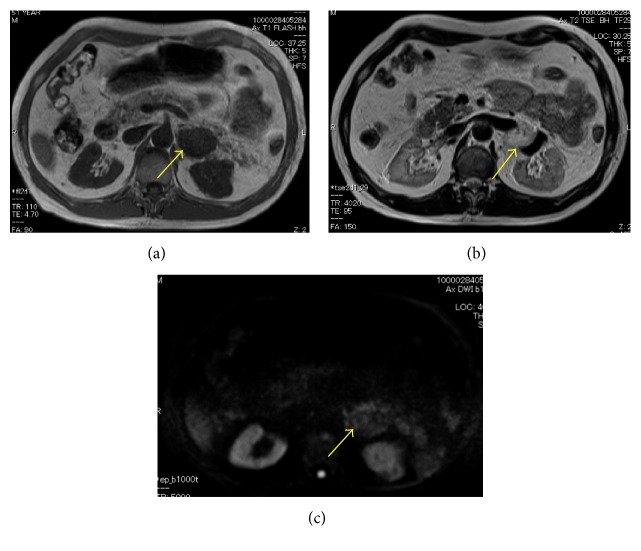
MRI images. (a) T1WI, (b) T2WI, and (c) DWI. The tumor (arrow) showed low intensity in T1WI and high intensity in T2WI.
